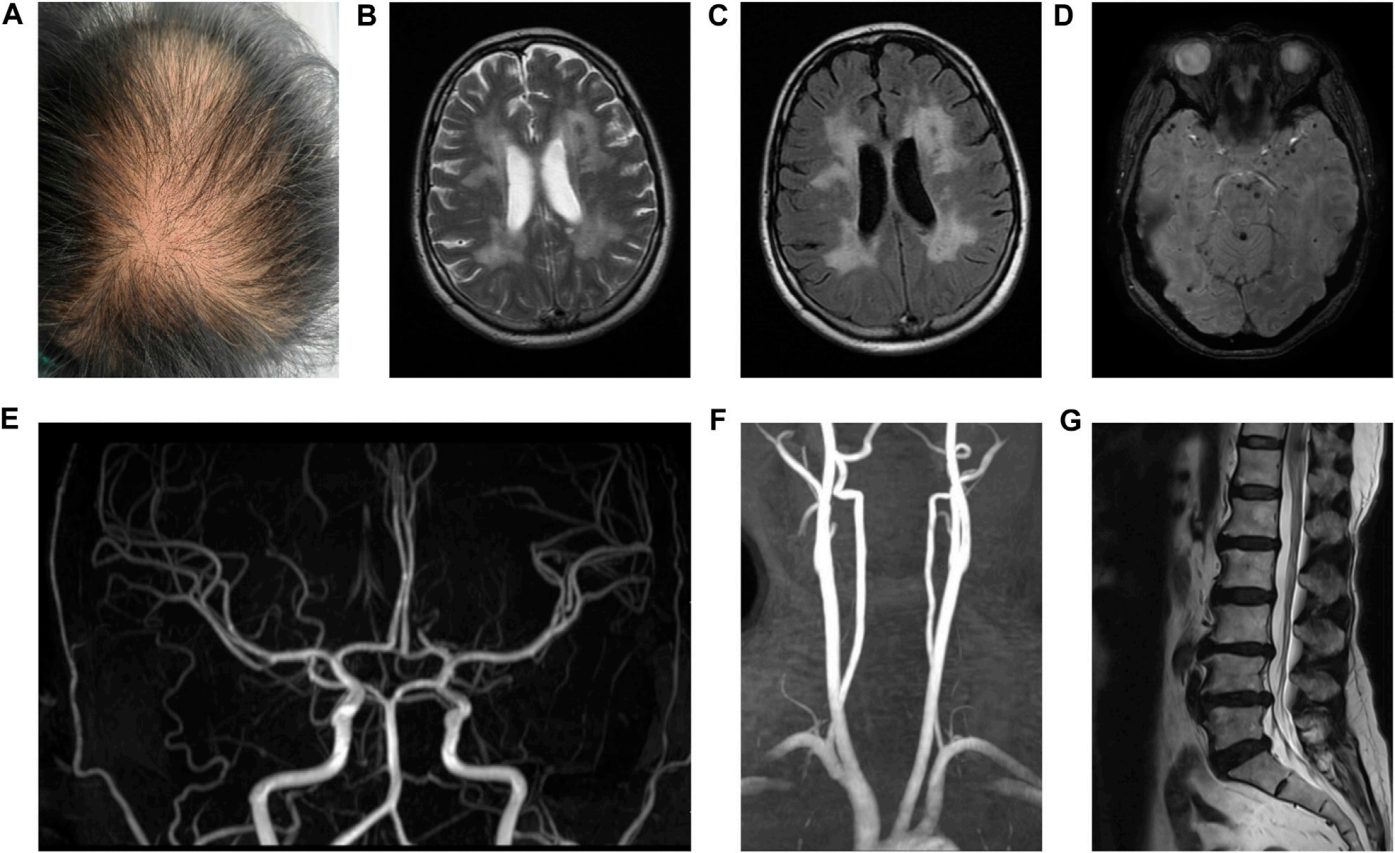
Hair and MRI of the patient. Hair of the patient (A). T2W and flair images revealed diffuse white matter lesions and ischemic infarctions (B and C). SWI showed microbleeds in the brain parenchyma (D). MRA showed normal intracranial arteries (E) and carotid arteries (F). T2W image showed lumbar disc herniation and degenerative lesions (G).
